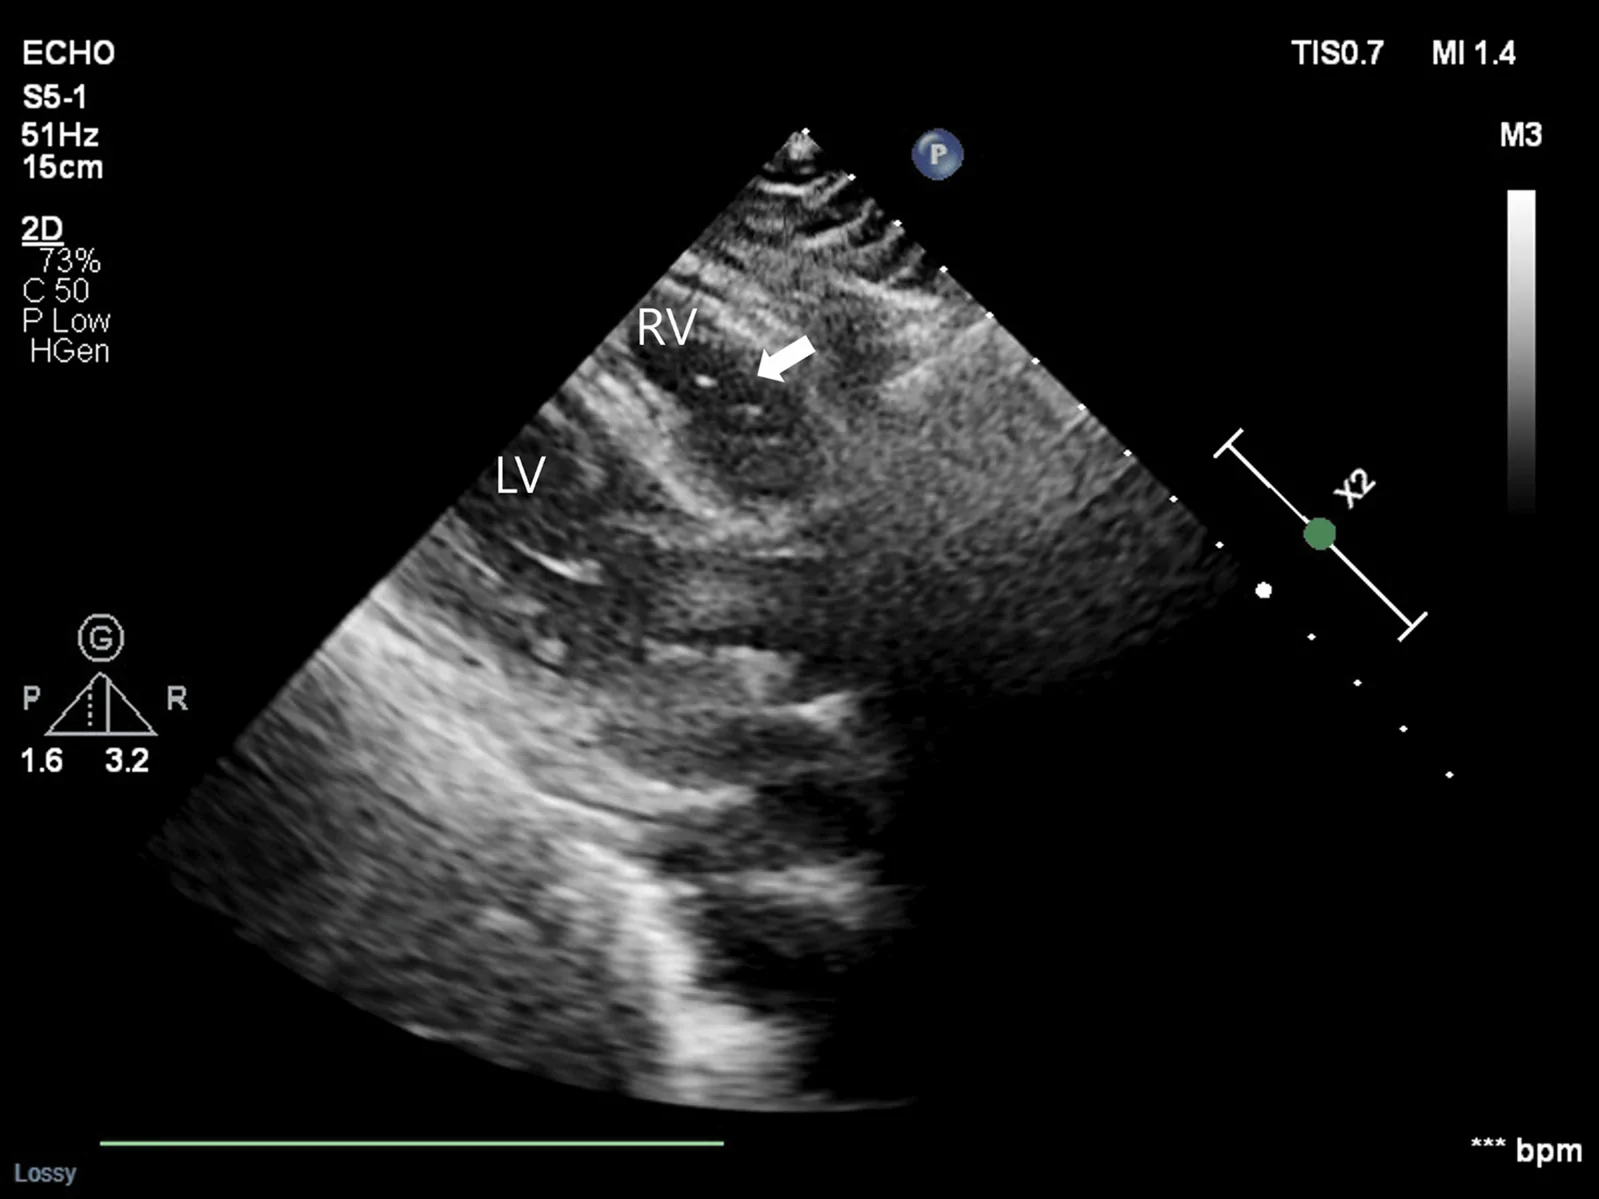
Transthoracic echocardiography demonstrating echogenic air bubbles within the right ventricle (white arrow) accompanied by transient right ventricular dysfunction.
